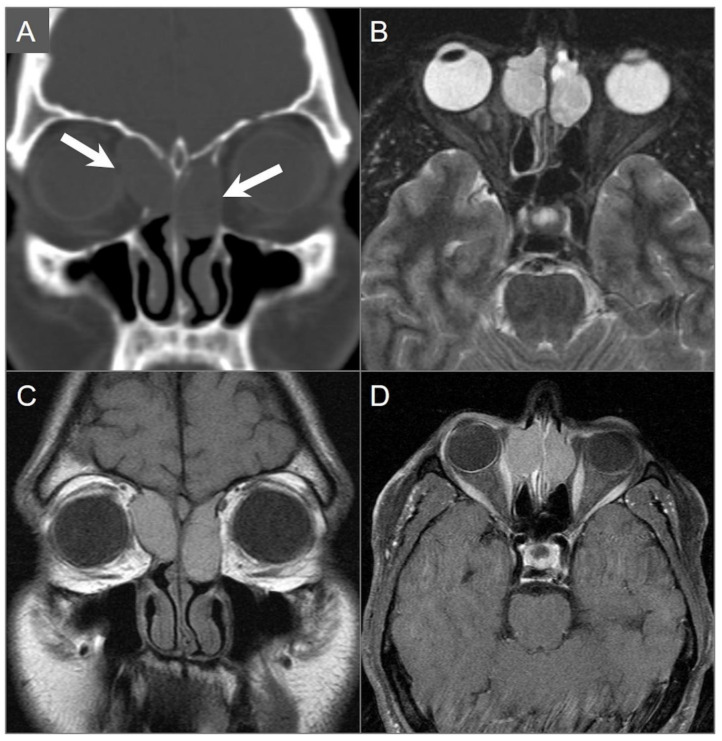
Mucocele. (A) Coronal CT image shows well-defined hypodense lesions in the bilateral ethmoid air cells with bony scalloping and dehiscence of the bilateral lamina papyracea. Additionally, note the extension into the orbits, which are greater on the right side (arrows). (B) Axial fat-suppressed T2W image shows moderate hyperintense signal in the lesions. (C) Coronal noncontrast T1W image demonstrates moderate corresponding hyperintense signal consistent with proteinaceous contents. (D) Axial postcontrast fat-suppressed T1W image demonstrates thin peripheral enhancement consistent with compressed mucosa. Mild mass effect on the right medial rectus muscle is noted.
